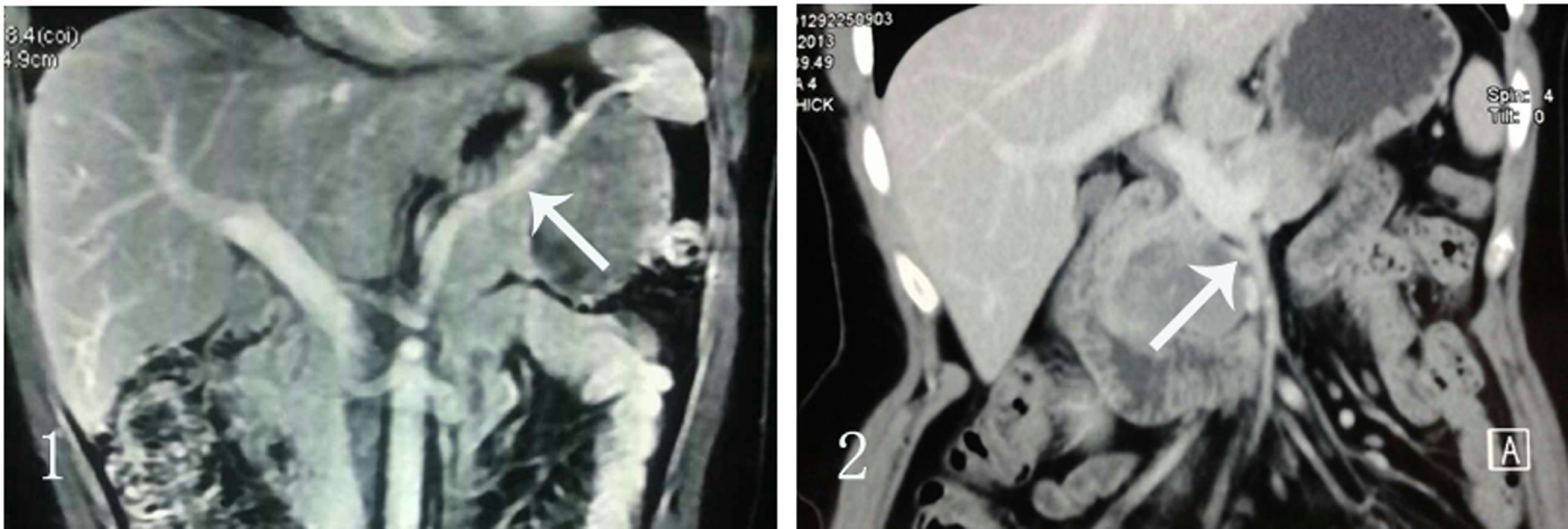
CT scan showed the vessels were jostled by the mass and had clear boundary. 1. The mass had clear boundary with spleen vessels. 2. The mass has clear boundary with SMV.
